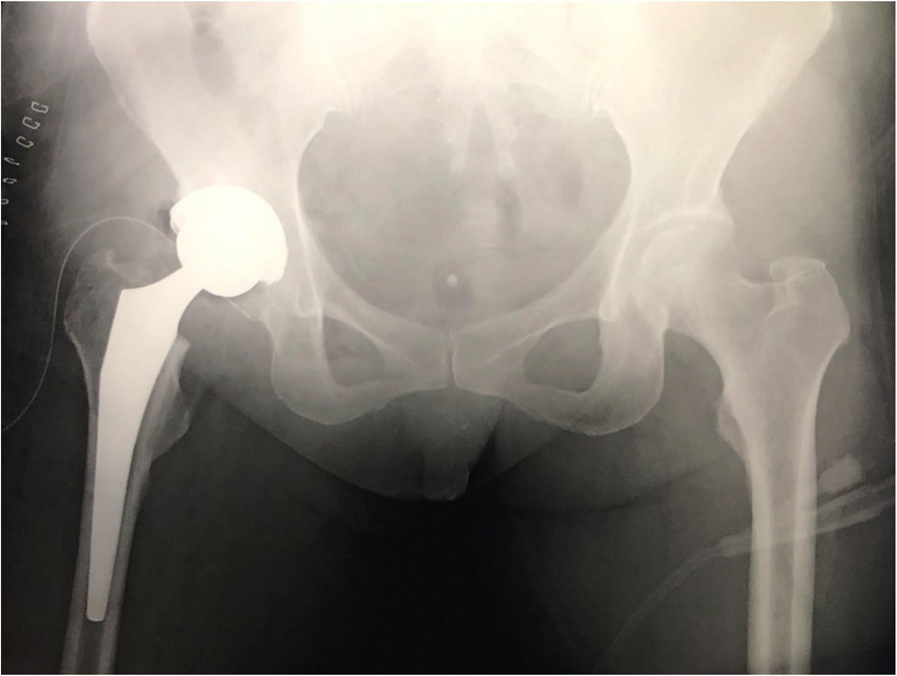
Anteroposterior plain radiography of both hips after total hip arthroplasty (THA)
